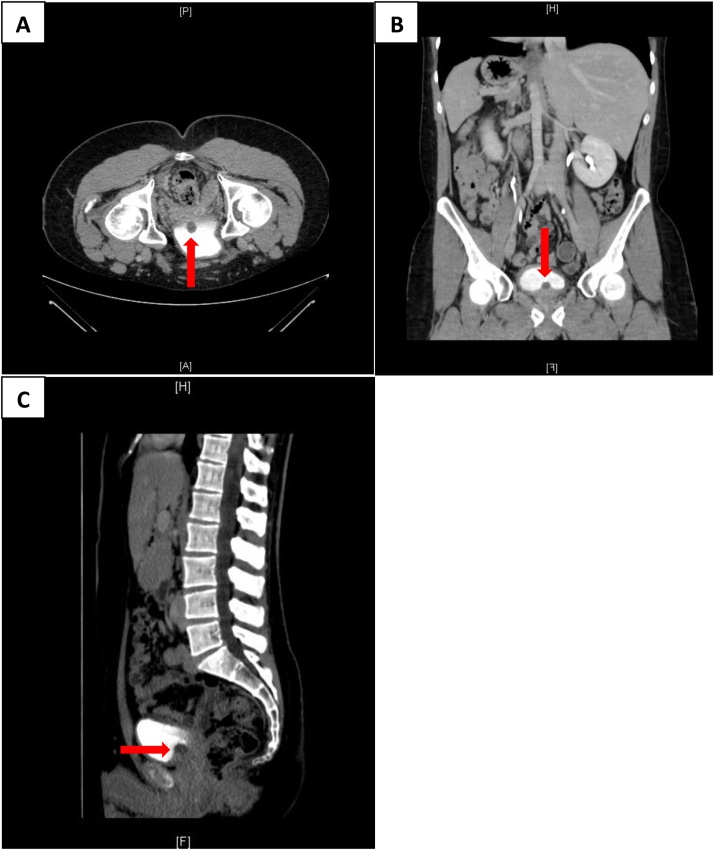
Axial (A), coronal (B) and sagittal (C) CT urography images showing a low-density cystic lesion measuring 1.5*1.4 cm in size at the midline of the upper part of the bladder neck region indicating an anteriorly positioned prostatic cyst (red arrow).
